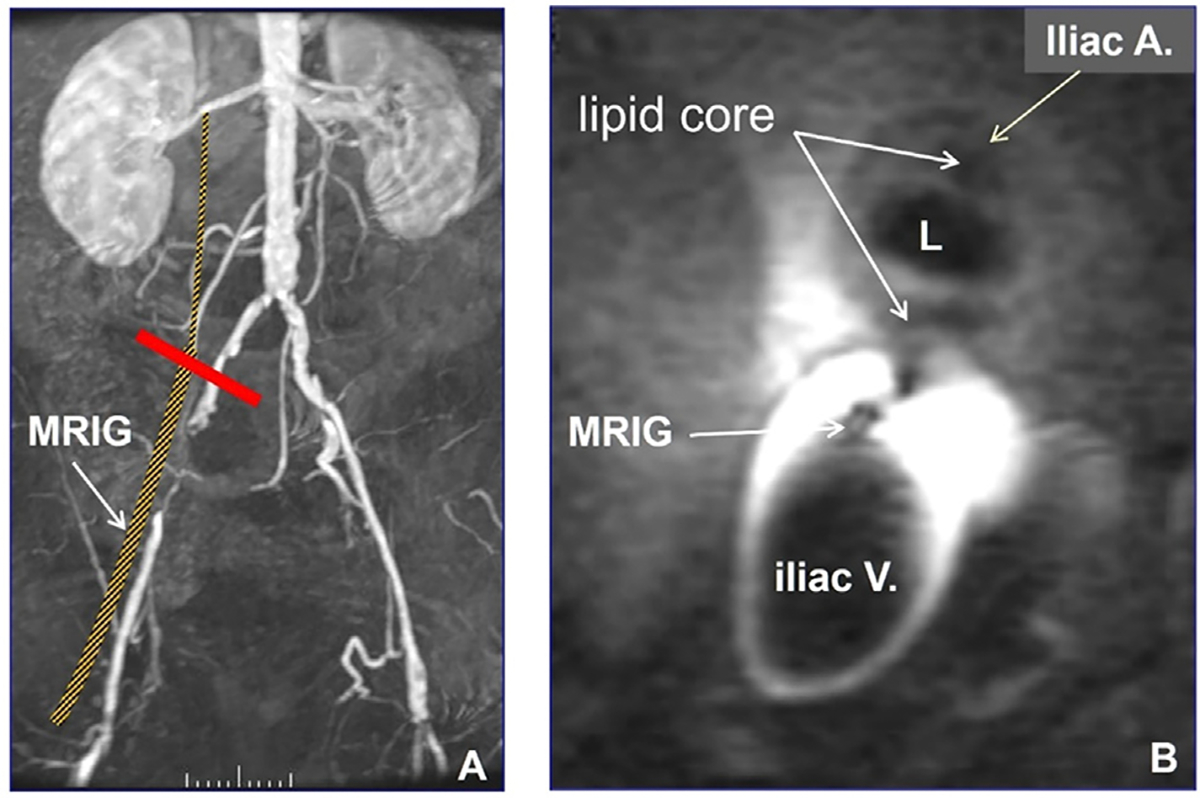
Transvenous high-resolution MRI of atherosclerotic plaque in an iliac artery. (A) The MR imaging-guidewire (MRIG) is positioned in the iliac vein to obtain a cross-sectional view (red line) of the adjacent iliac artery. (B) The transvenous cross-sectional MRI captures high MR signal with the MRIG and depicts an atherosclerotic plaque with a lipid core narrowing the iliac artery lumen (L) (Courtesy of Lawrence Hofmann).
